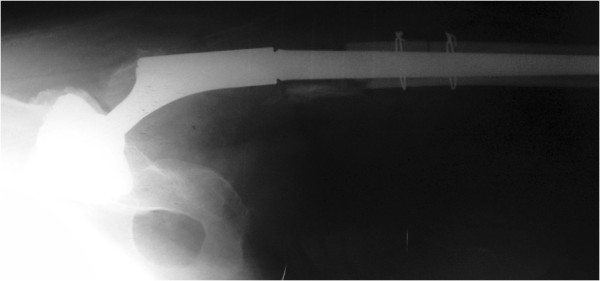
Postoperative plain radiograph. Note the excellent adaptation of the osteotomy lines.
